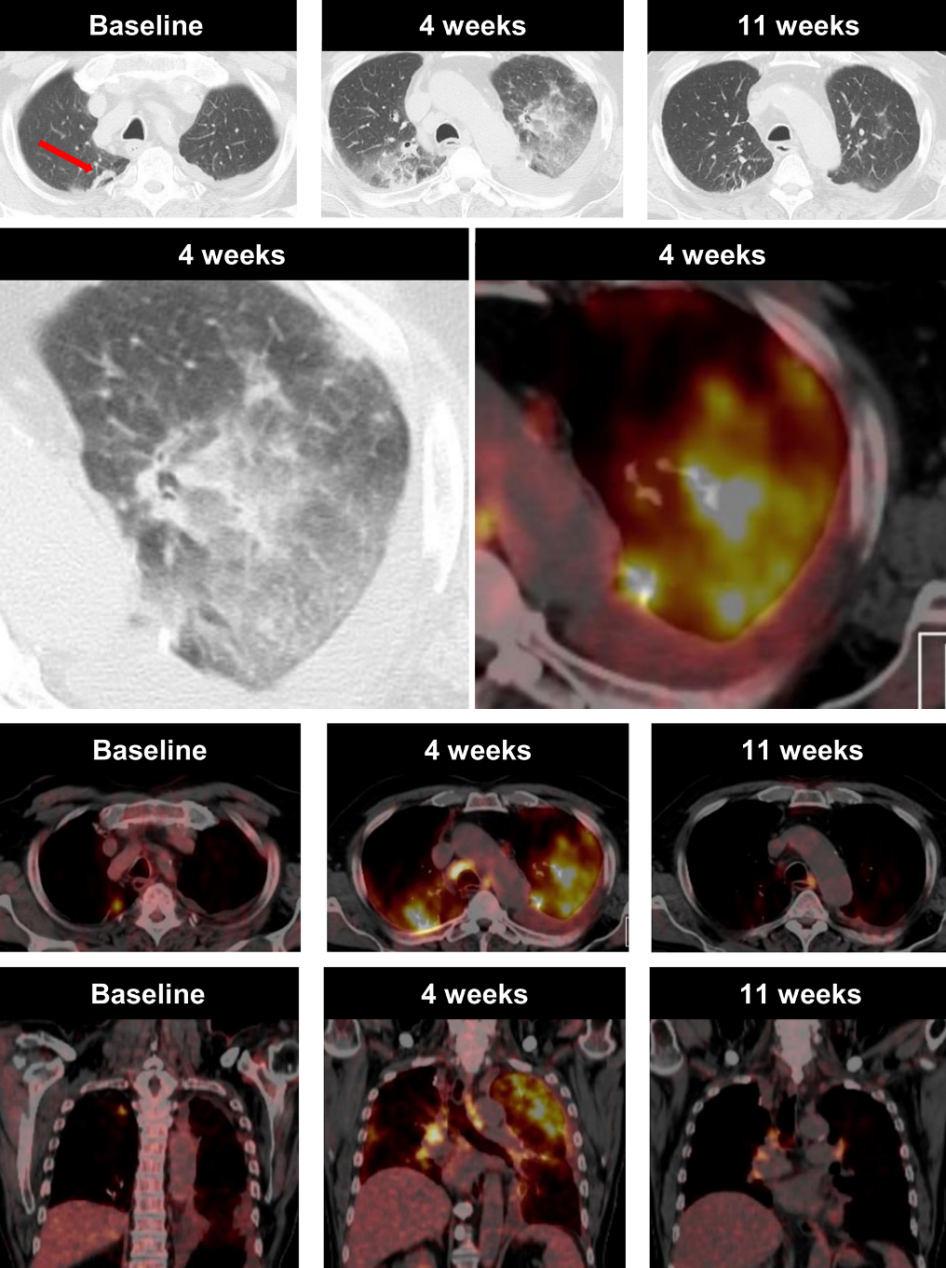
A case of pneumonitis in a 75-year-old male NSLCL patient, who was receiving immune checkpoint inhibitor therapy. Axial and coronal CT in lung window and fused ^18^F‑FDG-PET/CT imaging obtained before treatment initiation show a metabolically active metastasis in the right upper lobe, as well as five cerebral metastases (not shown). Four weeks after treatment initiation, the pulmonary, as well as the cerebral metastasis (not shown), showed a significant tumour shrinkage. In contrast, new, patchy, highly metabolically active ground-glass opacities in both lungs (upper lobes > lower lobes), as well as metabolically active enlarged mediastinal lymph nodes, were visible. On the day of the examination the treatment was discontinued and the patient was started on cortisone. After 7 weeks the pulmonary changes resolved almost completely while slightly enlarged metabolically active mediastinal lymph nodes remained. Upper three rows: axial view; lower row: coronal view
